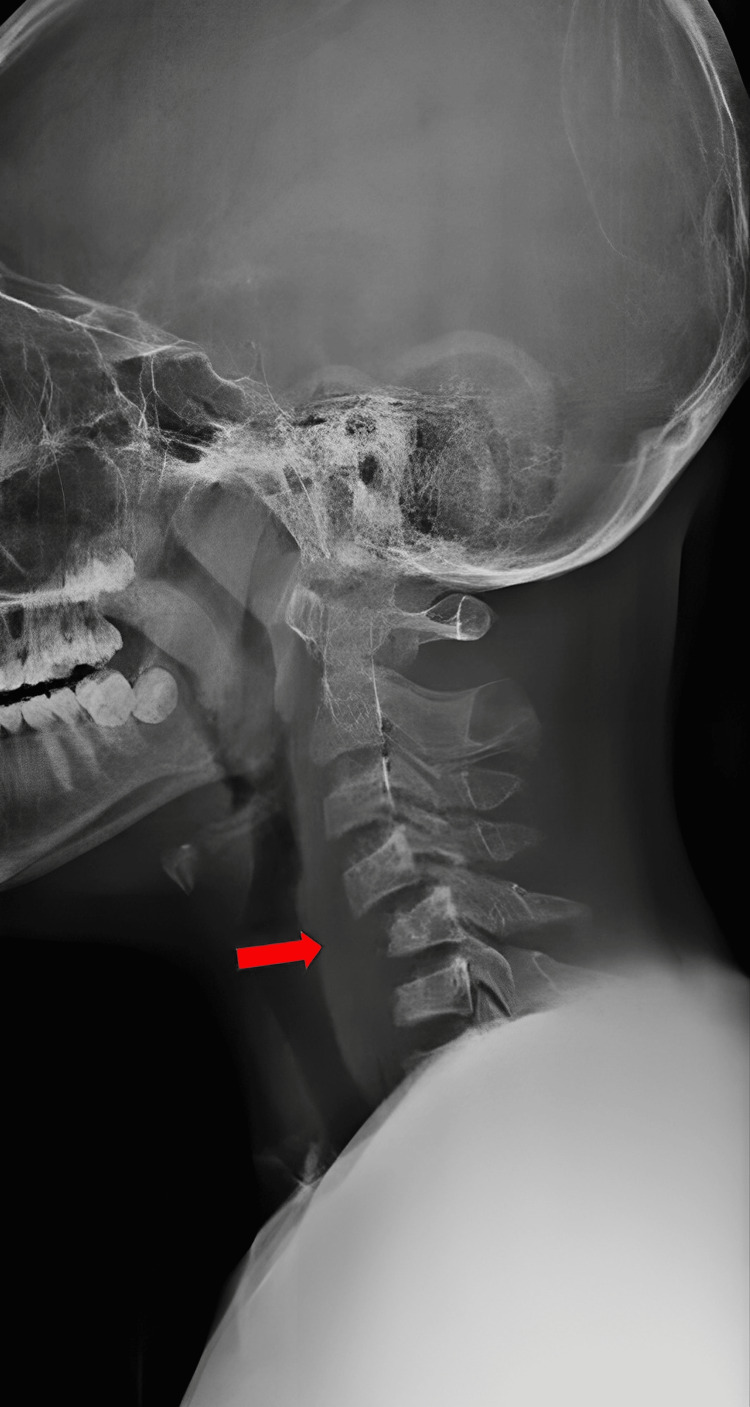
Lateral view of cervical X-ray with red arrow showing anterior translation of C4 over C5 with loss of cervical lordosis.
